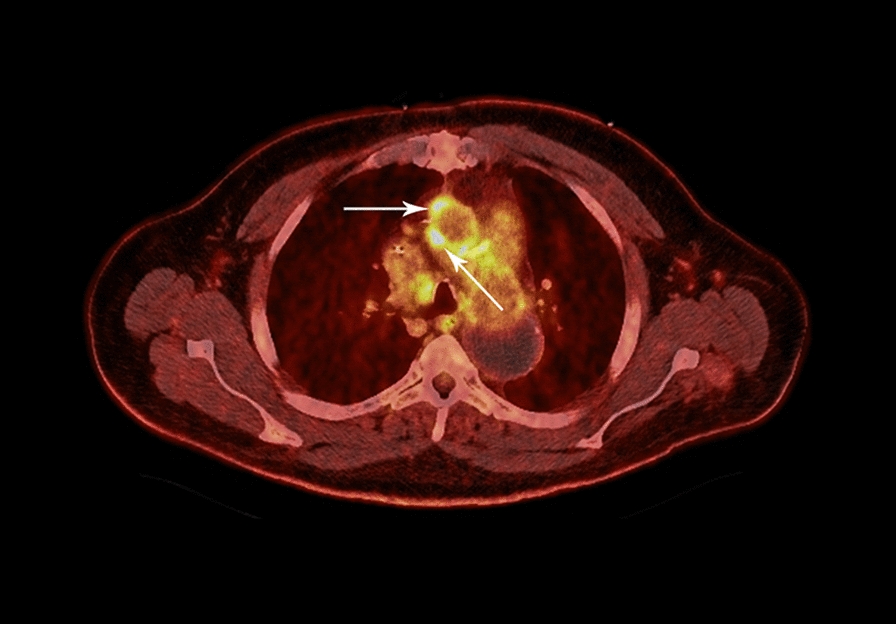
Positron emission tomography–computed tomography (PET/CT) of the chest. Hypermetabolic soft tissue (arrows) along the right lateral and anterior aspect of the ascending aortic endograft with a maximum standardized uptake value of 9.4, suspicious for aortic endograft infection
